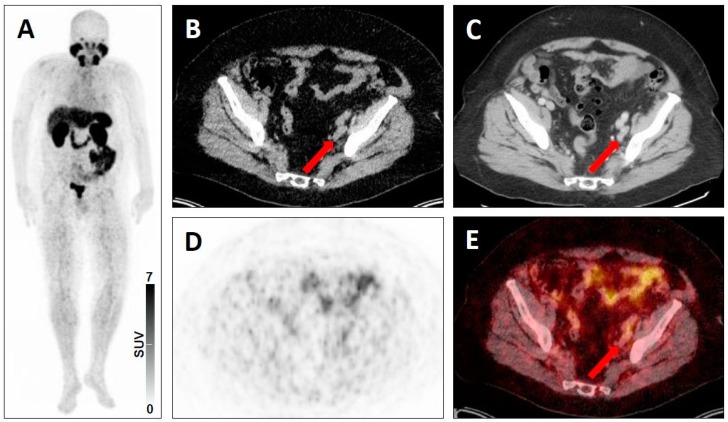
Equivocal uptake in soft-tissue lesion typical of prostate cancer lesion on PSMA-targeted positron emission tomography (PET)/computed tomography (CT). ^68^Ga-PSMA-11 PET/CT for restaging in a 73-year-old man with a history of a prostate cancer. Whole-body maximum intensity projection image showing absence of clearly pathologic radiotracer uptake (A). Axial low-dose CT (B), contrast-enhanced CT (C), PET (D), and fused PET/CT (E) showing an iliac lymph node typical of a prostate cancer lesion on anatomic imaging, but with equivocal uptake (red arrows), consistent with a PSMA-RADS-3A lesion.
